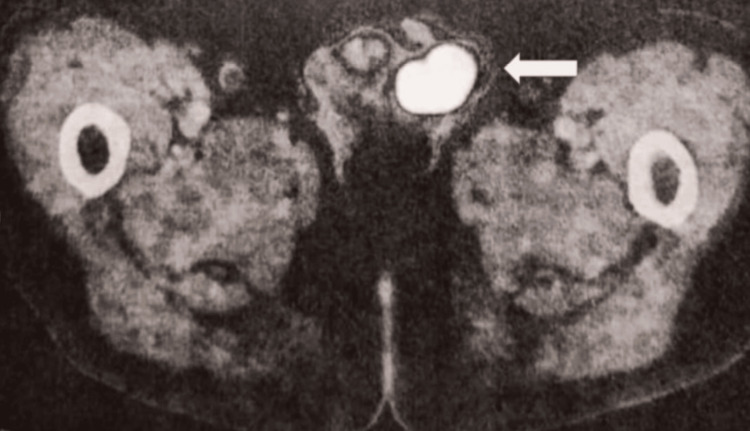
Body PET-CT with 18-fluorodeoxyglucose (FDG) axial image (arrow) shows a solid lesion in the left testicle, measuring 38 mm on the major axis.
